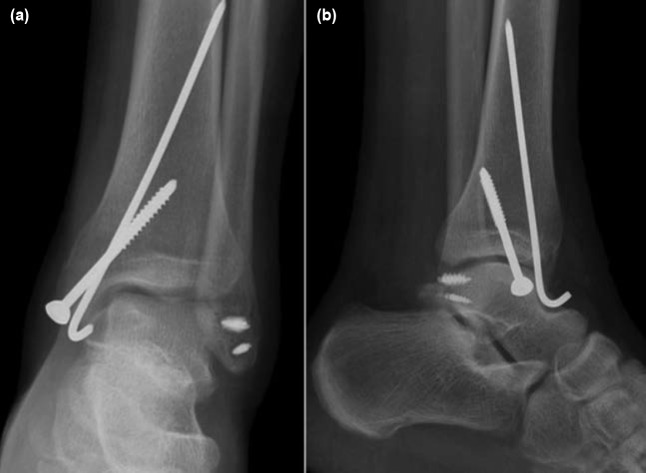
(a) Anteroposterior and (b) Lateral ankle radiographs of the patient six months after the surgery.
